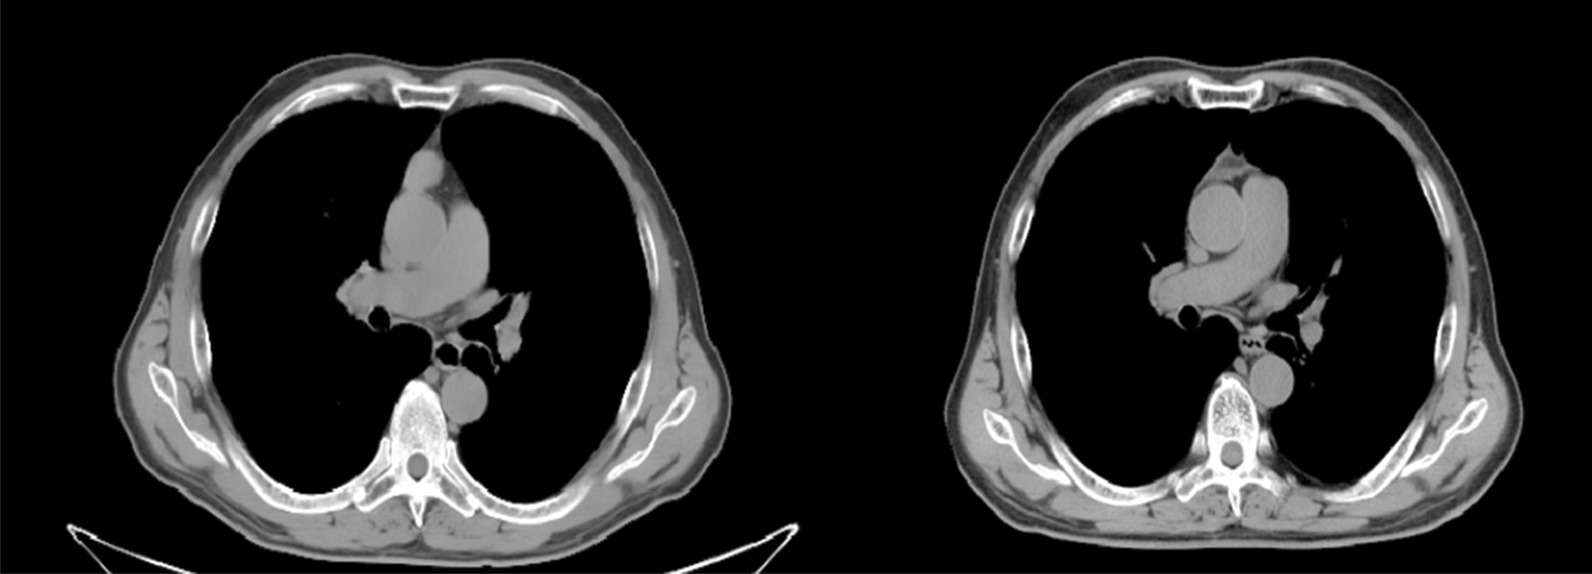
Left: In the anterior mediastinum, there was a soft tissue shadow of round shape, with a size of about 17 mm × 24 mm, and a computed tomography (CT) value of about 62 HU Right: One year after surgery, the chest CT showed the density of patchy fluid in the anterior mediastinum.
